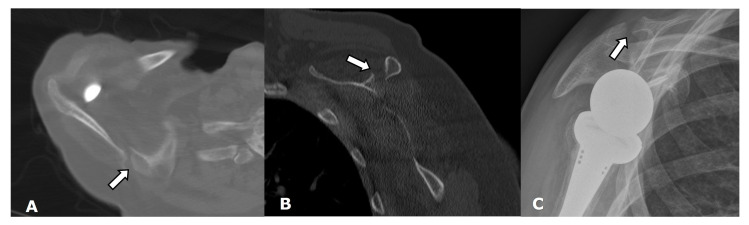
(A) Axial and (B) parasagittal CT images and (C) a scapular Y radiograph demonstrate a scapular fracture (arrow) following RTSA.
